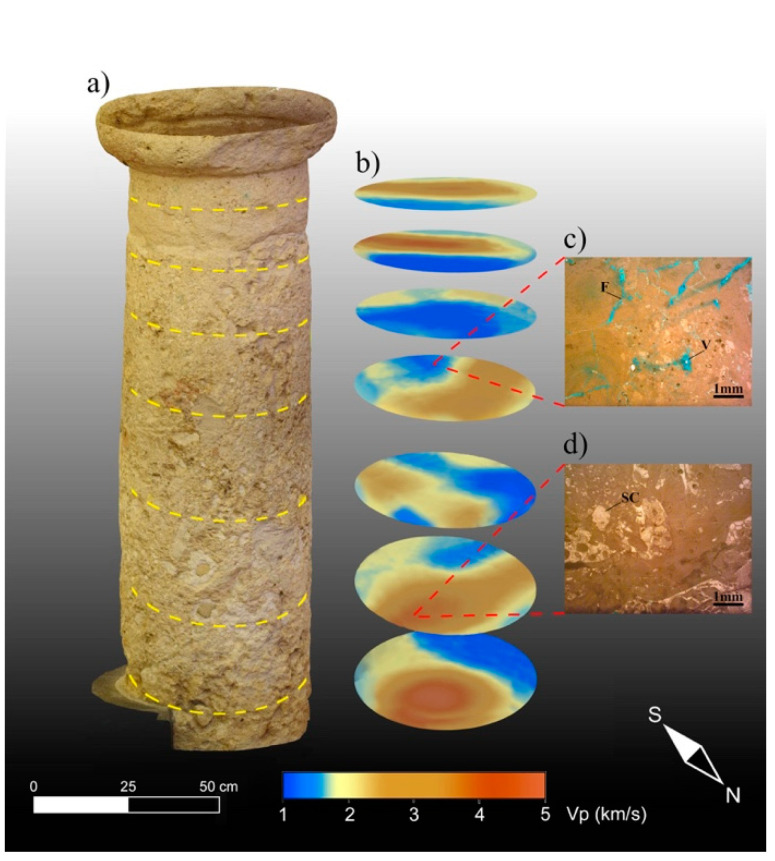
Integrated analysis of the ultrasonic tomography and thin sections for column 2. (a) CRP model; (b) tomographic slices; (c) thin section of the Pietra Forte in a sound sector of the shaft; (d) thin section of the Pietra Forte in a degraded sector of the shaft. F = fracture, V = vug, SC = sparry calcite cement.
